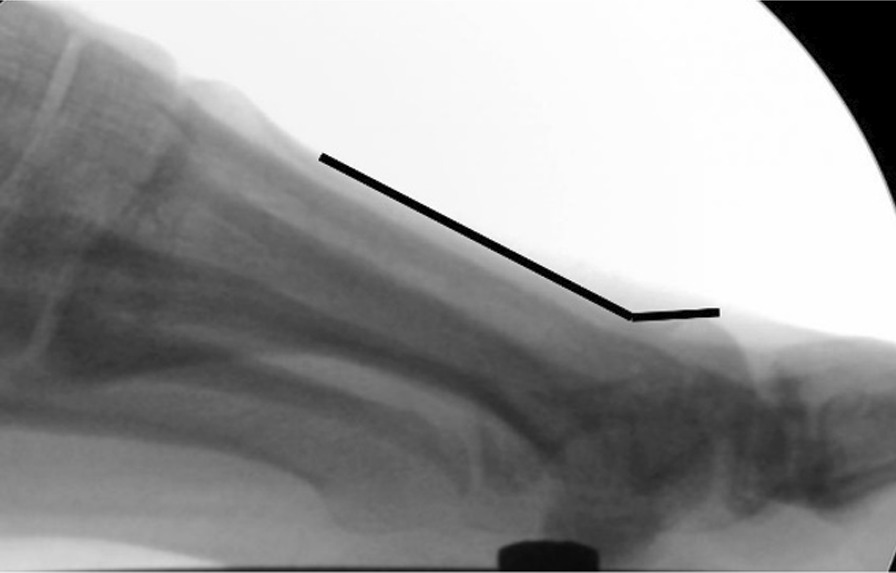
Measurement of metaphyseo-diaphyseal angle on lateral X-ray
